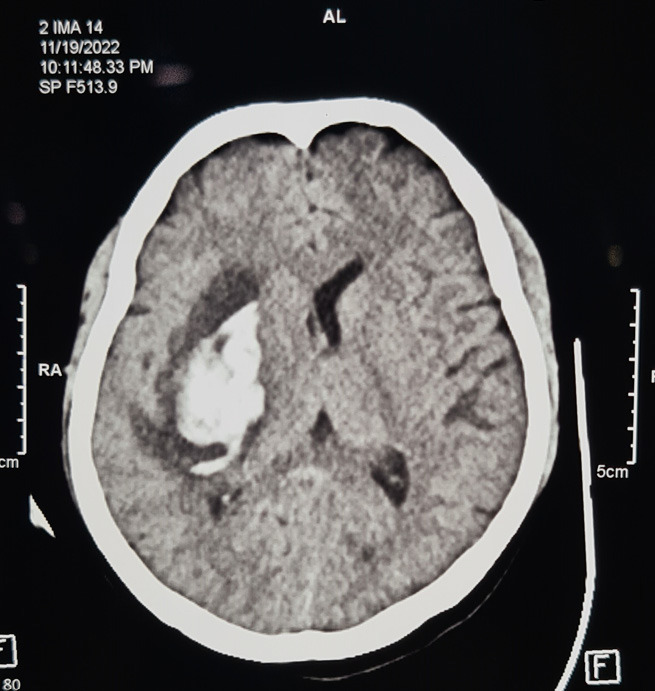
Noncontrast computed tomography head showing intracerebral haemorrhage with mass effect.
